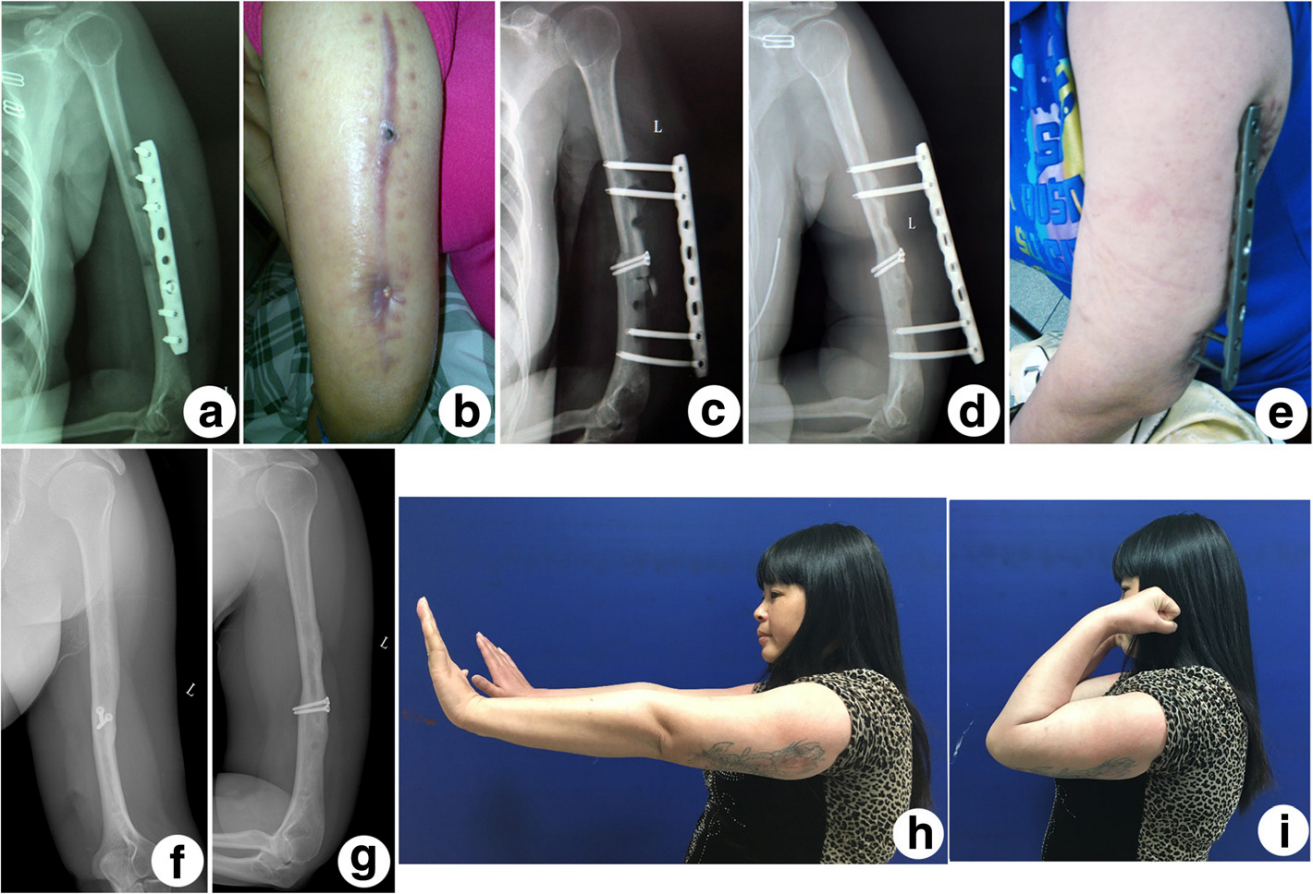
(a) X-ray of A 40-year-old female showing implant failure, nonunion at the 3-month after the first surgery. (b) Erythema, sinus and purulent discharge were seen in the affected upper limb. (c) Immediately postoperative X-ray showed the quondam LCP was applied as external fixator. (d) X-ray showing complete bone union at the 4-month follow-up. (e) Photograph showing the low profile plate. (f) Anteroposterior and (g) lateral X-ray at the last follow-up showing a full bony healing with an acceptable alignment. (h) and (i) Photographs at the last follow-up showing excellent function of the elbow, recovery of radial nerve function and absence of erythema, sinus or purulent discharge of the affected upper limb
